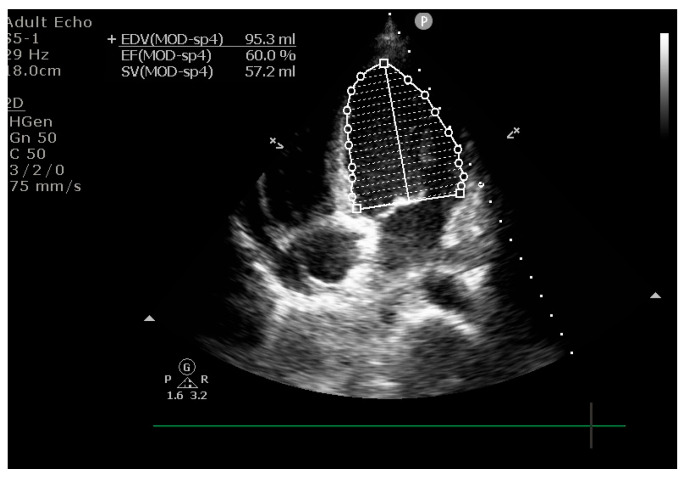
Echocardiographic findings showing an LVEF of 60%.
